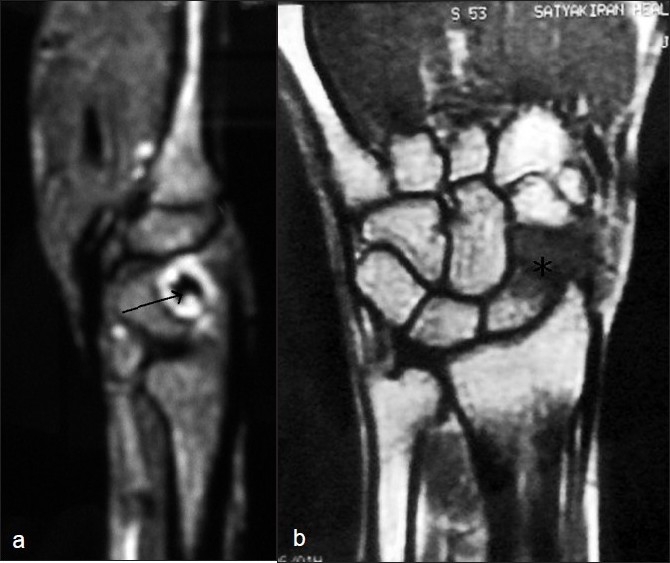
(a) MRI sagittal section through volar part of wrist showing osteonecrosis of distal pole of scaphoid (arrow): (b) MRI coronal section through mid part of the wrist showing osteonecrosis of distal pole of scaphoid (asterisk)
